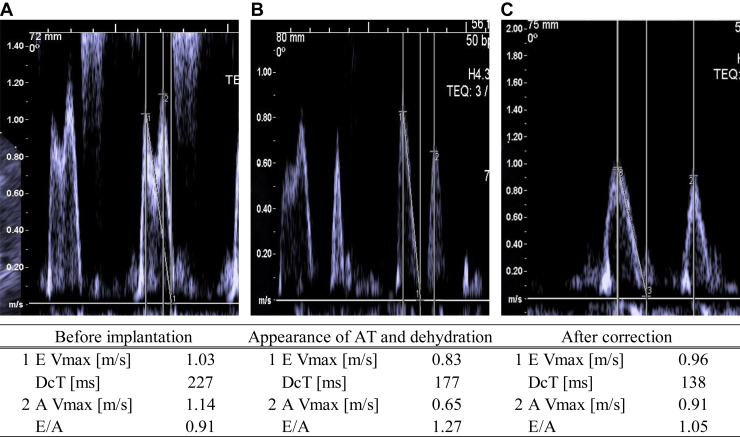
Evaluation of E/A ratio by echocardiography. A: Before Micra AV (Medtronic) implantation. B: Appearance of atrial tachycardia and after dehydration. C: After correction for dehydration by intravenous infusion. AV = atrioventricular; E/A = Emax/Amax.
